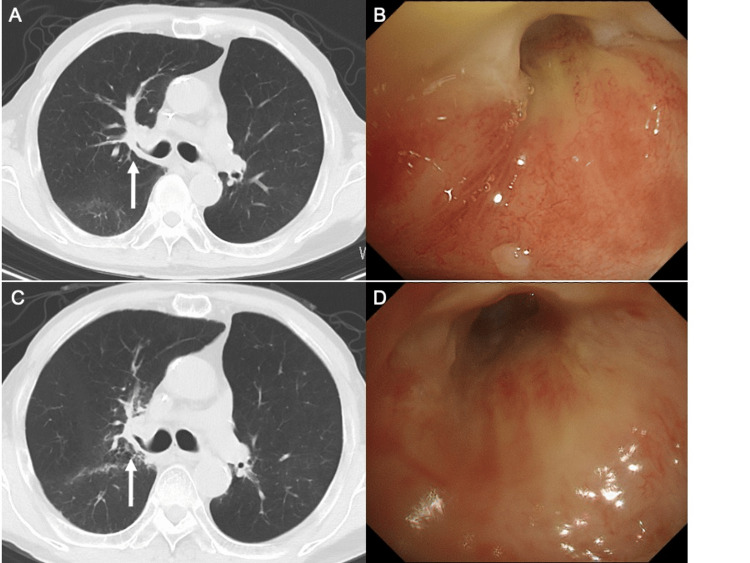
Chest computed tomography shows tumor shrinkage (white arrow) equivalent to a partial response at the end of the fourth cycle of combination chemotherapy (A), with tumor shrinkage (white arrow) maintained at the end of the eighth cycle of combination chemotherapy (C). Bronchoscopic image shows patency of the orifice of the right upper lobe bronchus at the end of the fourth cycle of combination chemotherapy (B), and slight improvement of patency at the end of the eighth cycle of combination chemotherapy (D).
